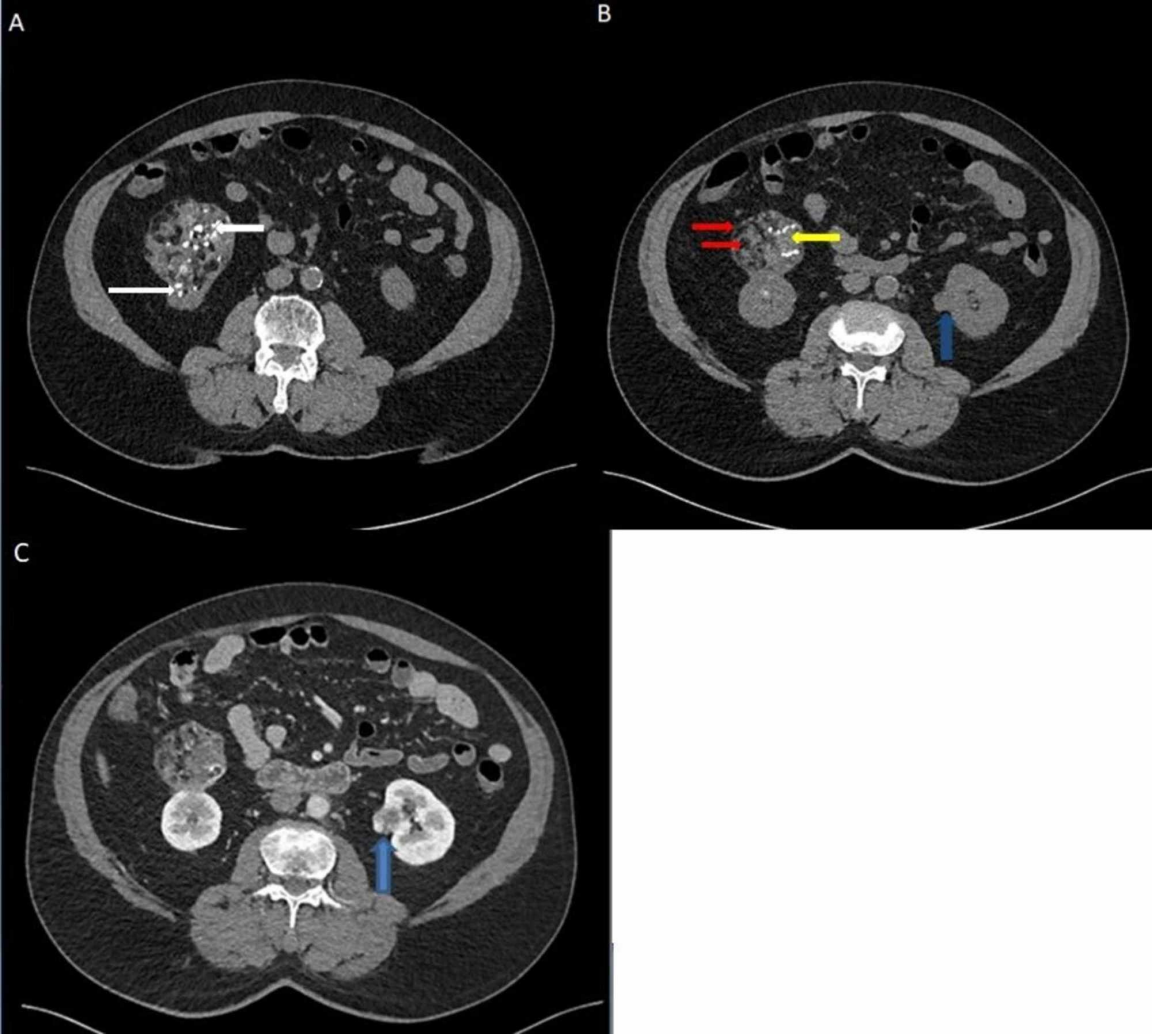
Axial sections of CT of the abdomen and pelvis at the level of lower poles of the kidneys A large exophytic fat-containing heterogeneous mass with focal calcifications (white arrows) in the lower pole of the right kidney, measuring 77 x 61 mm, can be seen (A-C). The hypodensities (red arrows) may explain the fatty contents, whereas the density of the other parts (yellow arrow) of the tumor is similar to the right kidney. A perceived heterogeneous faint contrast enhancement can be seen in the right kidney lesion (C). A suspicious small exophytic renal nodule measuring 14 mm in diameter is seen at the lower pole of the left kidney as well (blue arrow in 4B), showing heterogeneous enhancement (blue arrow in 4C).
